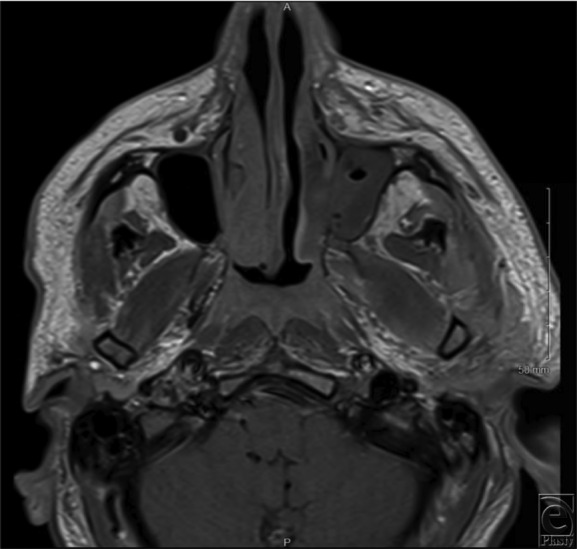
Admission T1-weighted magnetic resonance image; mucormycosis invasion along the left inferior turbinate and left maxillary sinus.
